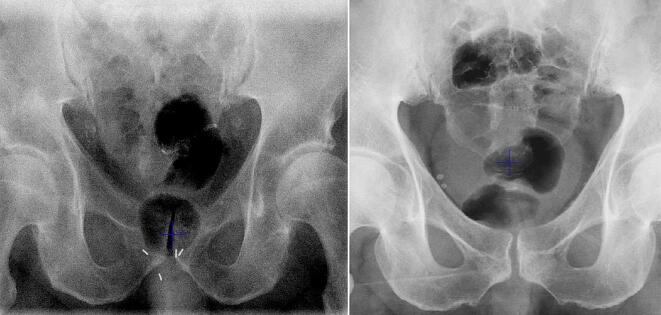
AP-kV images of two prostate patients exposing the bladder shadow, rectal gas bubbles, and the bony anatomy. In patient 1 (Left image), the AP-kV revealed a clinically relevant rectal gas posterior to the fiducial markers. As a result, RTTs decided to remove the patient from the bed before proceeding to CBCT. In patient 2 (Right image), the AP-kV exposed both the bladder and bowel gas, but RTTs decided to proceed to CBCT as the gas was located superiorly and far from the PTV.
